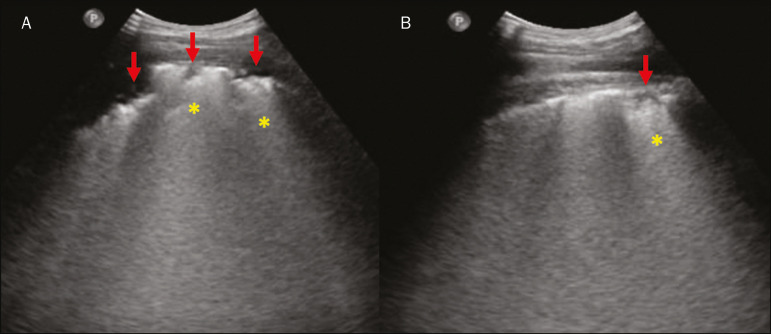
A,B: The chest ultrasound in a patient with COVID-19 demonstrates round small, hypoechogenic, and irregular subpleural consolidations (arrows). Pleural C-lines (asterisks) start from their posterior extremities, which are hyperechogenic and vertical, similarly to B-lines.
